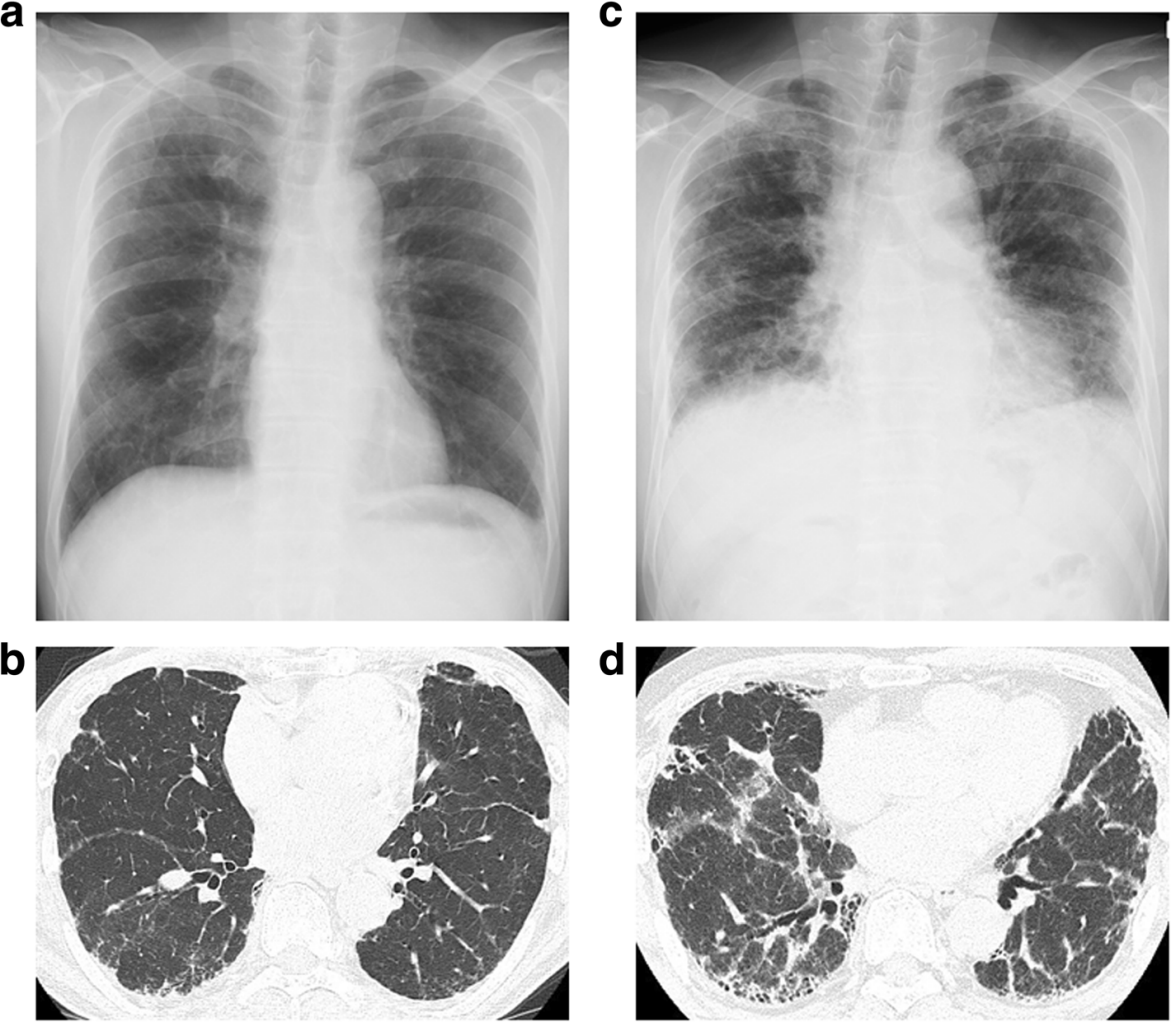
Chest X-ray and HRCT findings in 2013 (panels a and b) and 2018 (panels c and d). Both examinations revealed reticular opacities in the subpleural regions that progressed over the course of 5 years and were associated with a markedly reduced lung volume
